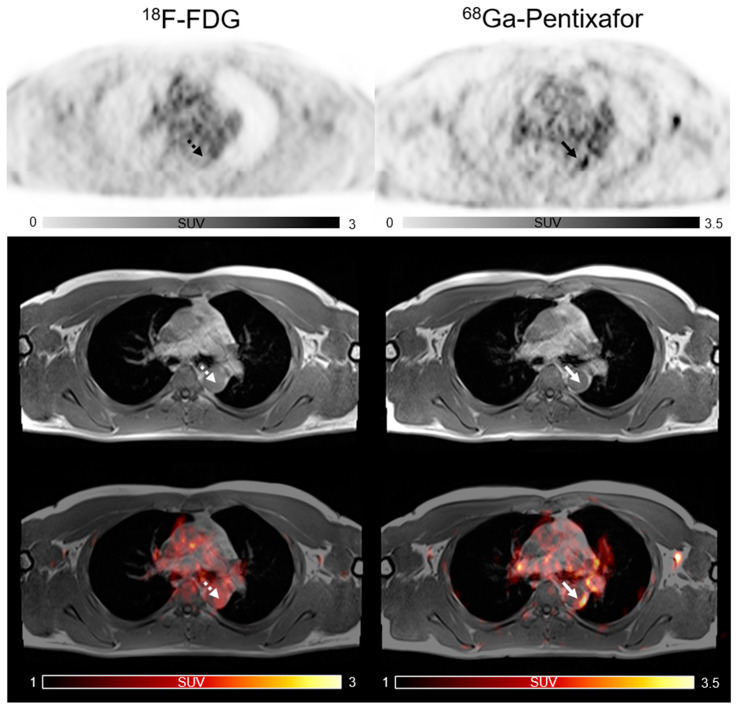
Focal vascular uptake of [^68^Ga]PentixaFor (white arrow) but without corresponding focal [^18^F]FDG uptake in active lesions (white dotted arrow).
